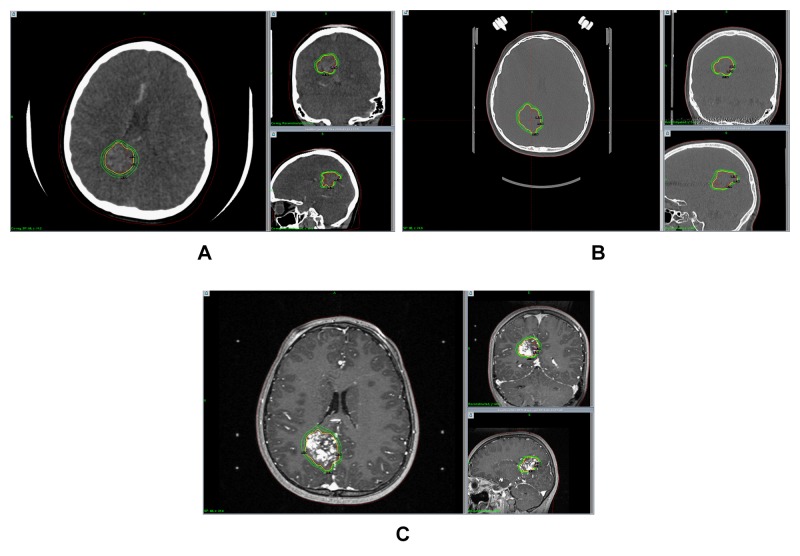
Sequential target delineation and contouring for GKRS treatment. The DICOM source volume images from the earliest filling phase of the 4D-CTA are used for contour development in GammaPlan® (A). On the day of service, the 4D-CTA, and the associated target contour, are co-registered to the volumetric CT head (B). The postgadolinium T1 and time of flight (TOF) MRI sequences are used for contour verification and further refinement as needed (C).
